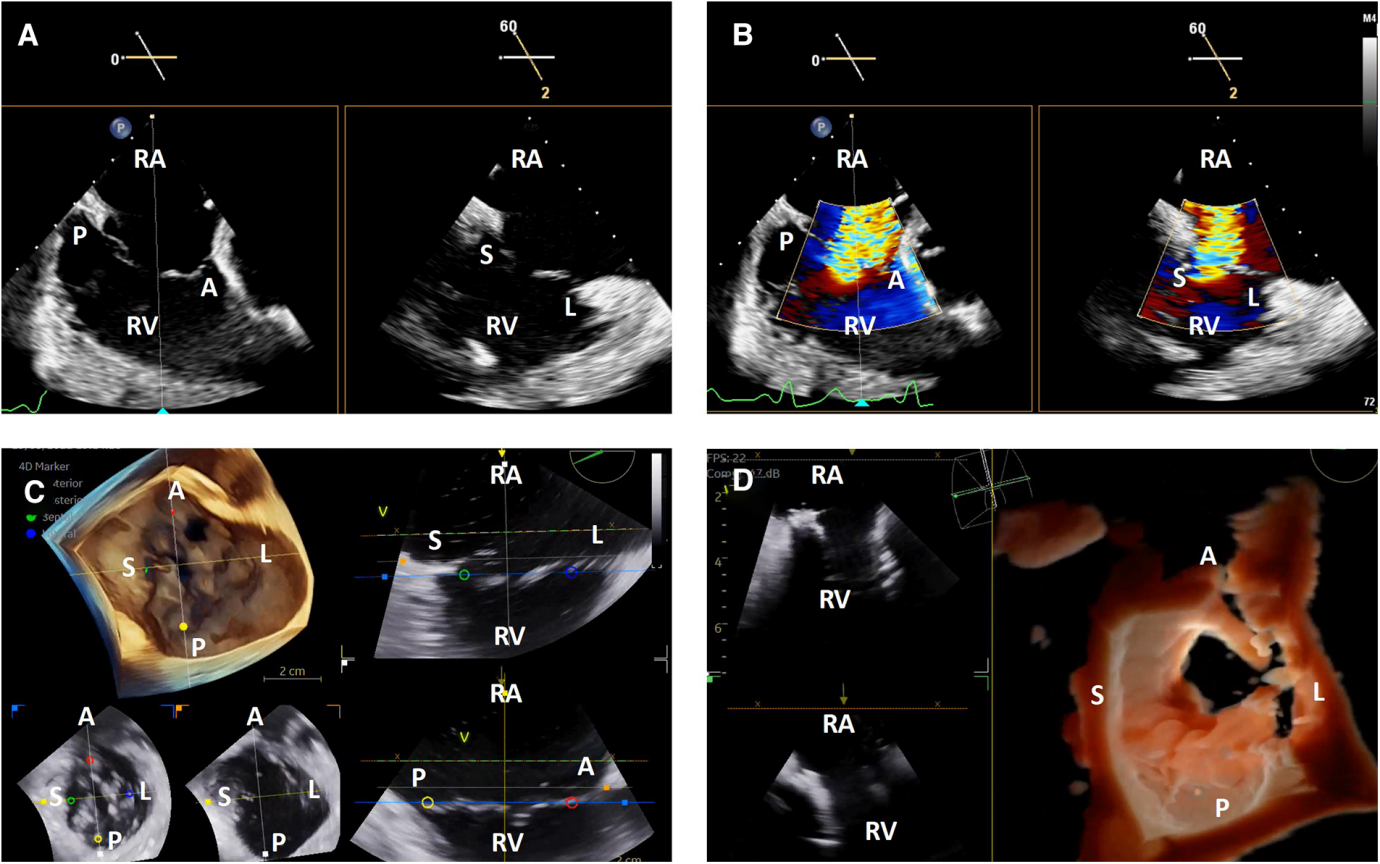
3-dimensional intracardiac echocardiography for transcatheter tricuspid interventions. Biplane intracardiac echocardiographic evaluation of the tricuspid valve without (A) and with (B) color Doppler; (C) live 3-dimensional multi-planar reconstruction of the tricuspid valve with color-coded anatomic markers and two independently controlled short axis views on the bottom left (leaflet tip level on the left, annulus level on the right); (D) 3-dimensional *en face* view of a degenerated prosthetic tricuspid valve in diastole with two simultaneous 2-dimensional image plane reconstructions, obtained during a tricuspid valve-in-valve implantation. RA, right atrium; RV, right ventricle; A, anterior; P, posterior; S, septal; L, lateral.
